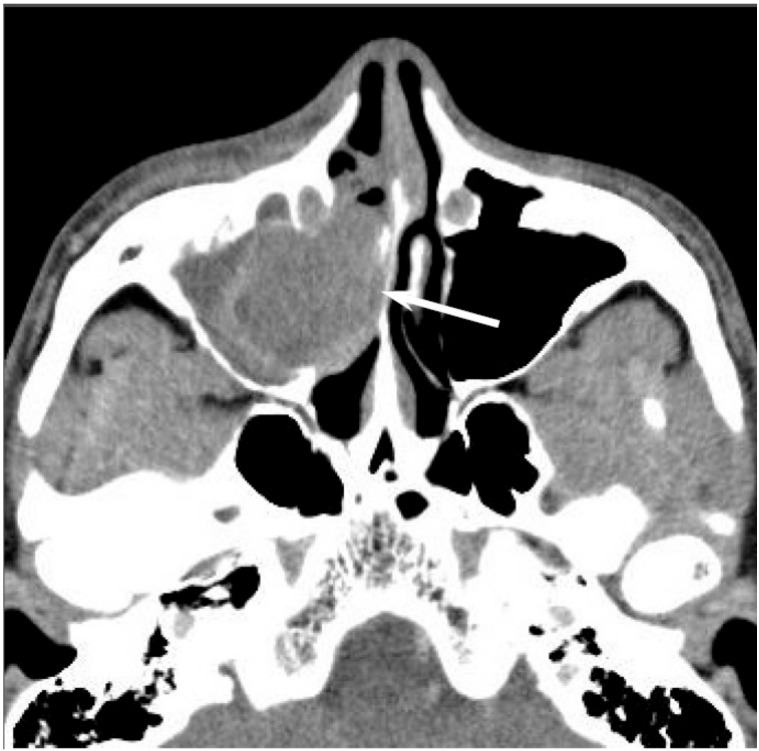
Rhabdomyosarcoma of the right nasal cavity. Contrast-enhanced CT image showing a homogeneously, slightly enhanced lesion (arrow).
